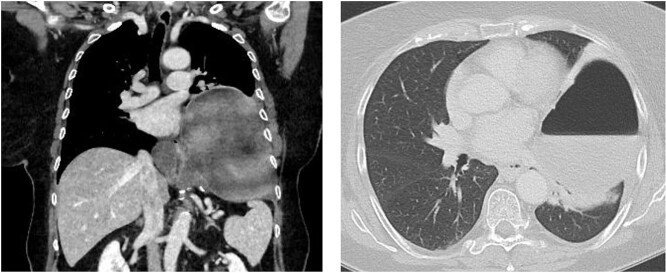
Chest/abdomen computed tomography showing a new recurrence with complete up-side-down stomach and compression of the left lower lung lobe.
